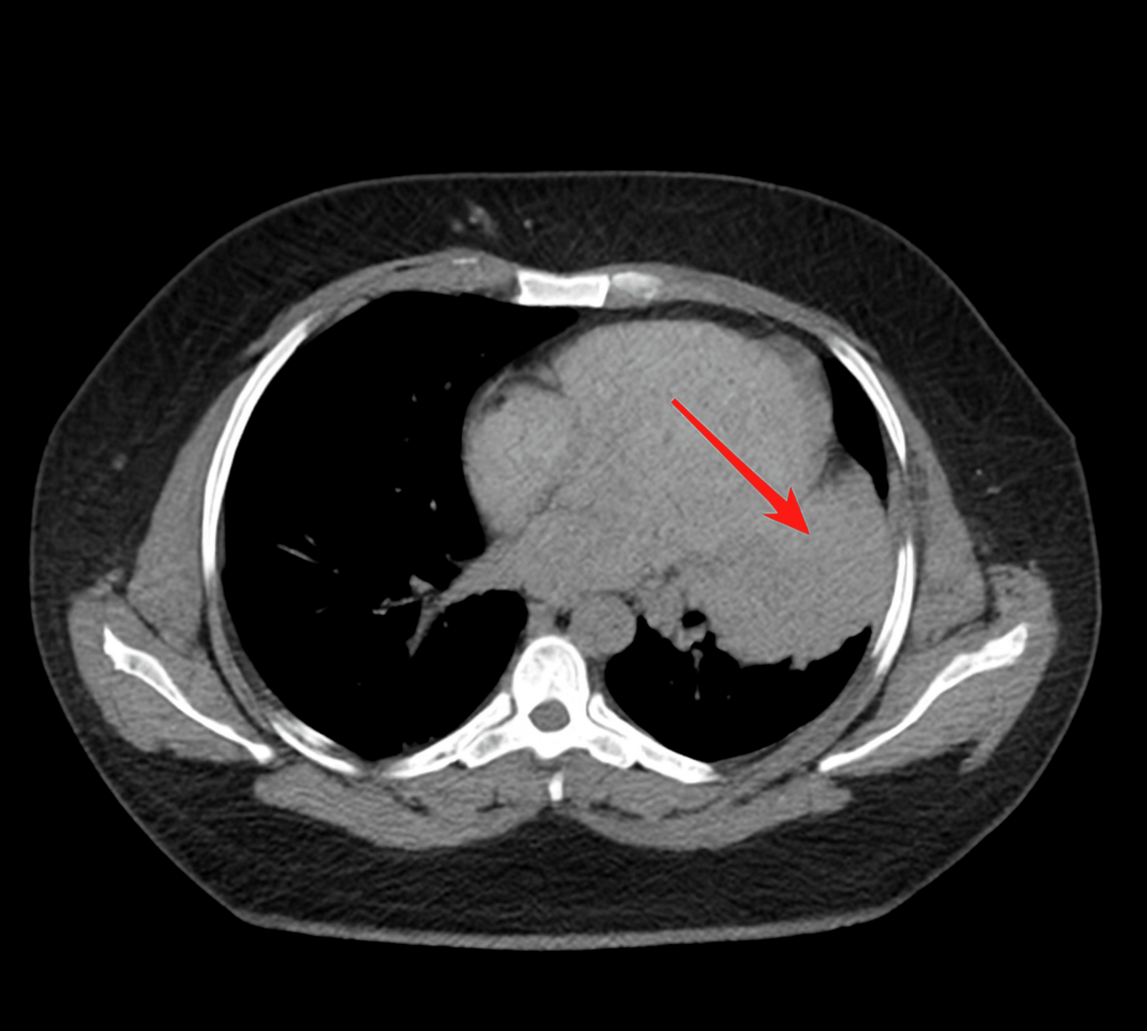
CT chest performed in March 2023 The image shows a left upper lobe (LUL) mass (red arrow).
